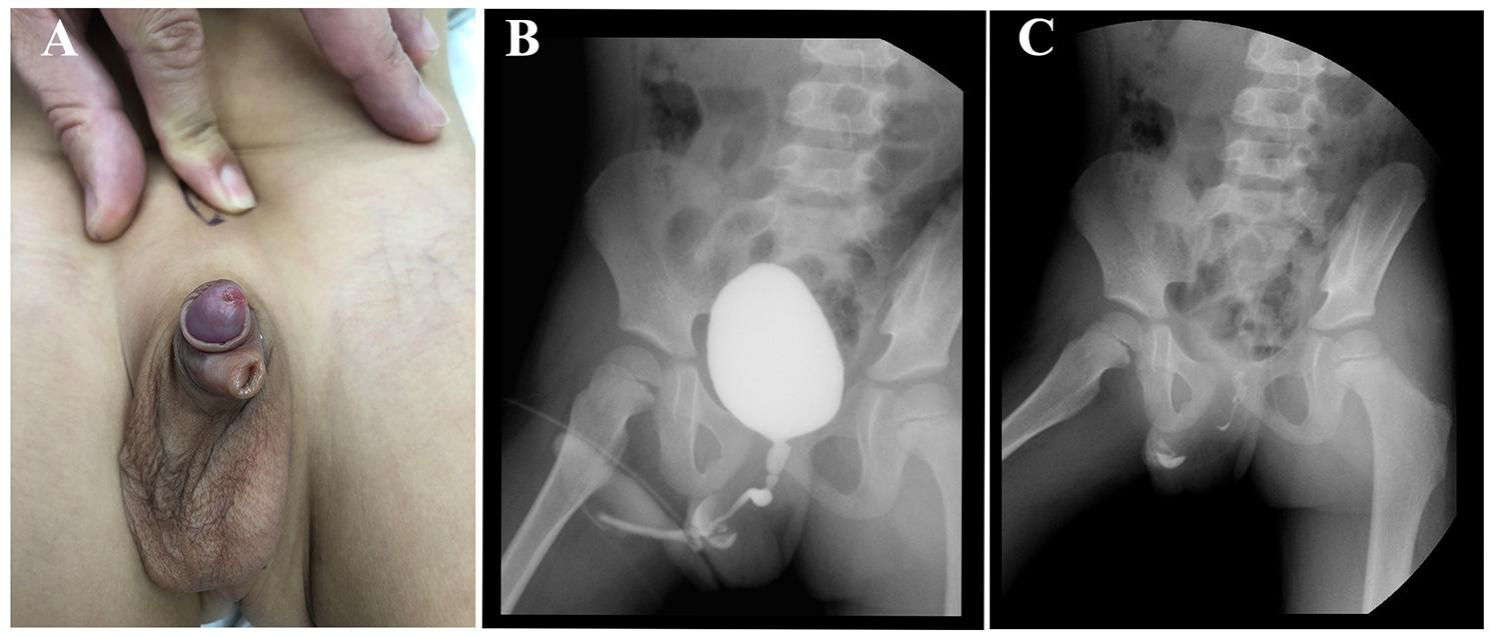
Preoperative clinical and imaging evaluation. (A) Clinical photograph showing a ventral, skin-covered appendage and approximately 90° counterclockwise penile torsion with an eccentric meatus. (B) Voiding cystourethrogram (VCUG), filling/early voiding phase: normal bladder contour with opacification and a single urethral channel; no duplicated tract is seen. (C) VCUG, post-void/late-phase plain film: no secondary urethral tract or fistulous communication is demonstrated.
